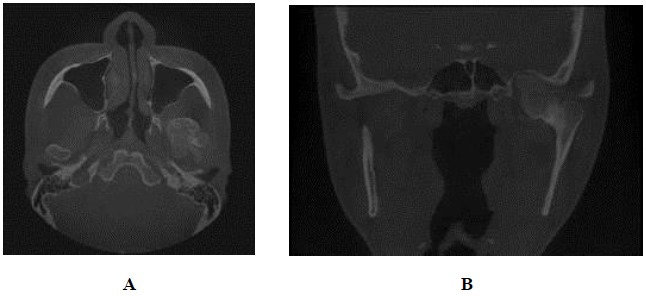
A, B Axial and coronal CBCT images showed a large bony mass arising from the left mandibular condyle extending anteriorly, posteriorly, medially and superiorly to the temporal bone. The lesion density was continuous with the structures of mandibular condyle.
